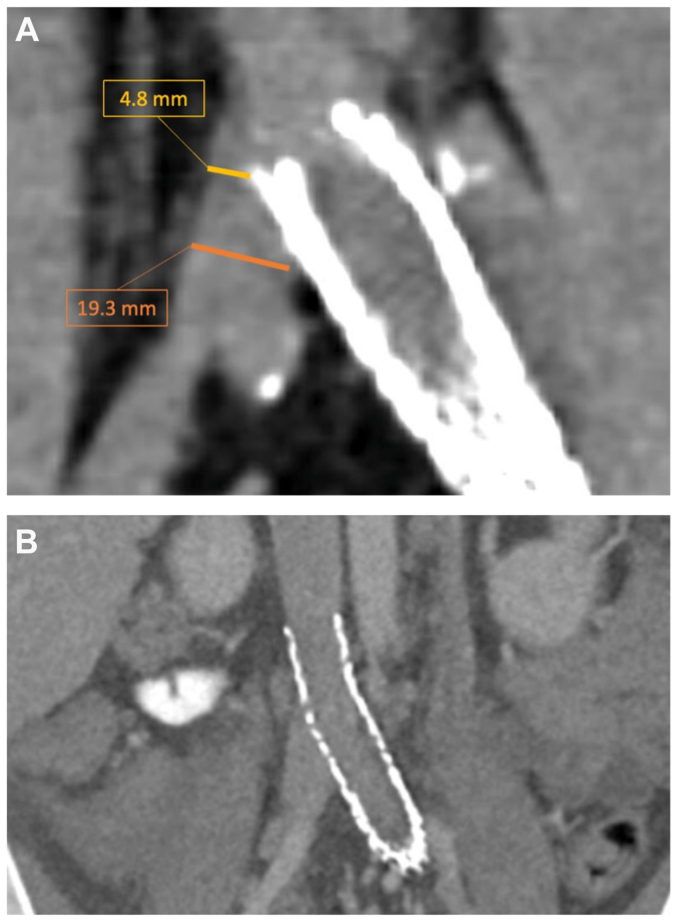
Determination of degree of overlap of contralateral iliac vein orifice on computed tomography (CT) scan. A, Total right iliac diameter just below confluence (19.3 mm) and unobstructed length of iliac vein at confluence (4.8 mm) yields calculation of 75.1% overlap. B, 100% overlap of contralateral iliac vein orifice with stent placed to opposite wall of inferior vena cava (IVC).
